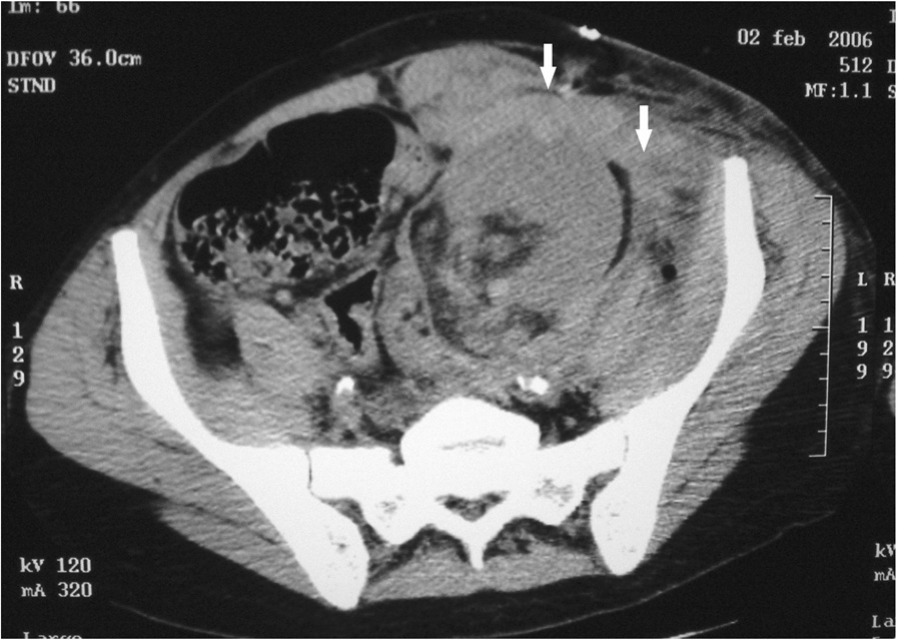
Abdomen and pelvis CT scan showing a massive retroperitoneal haematoma with active bleeding from the transplanted kidney (arrows)
